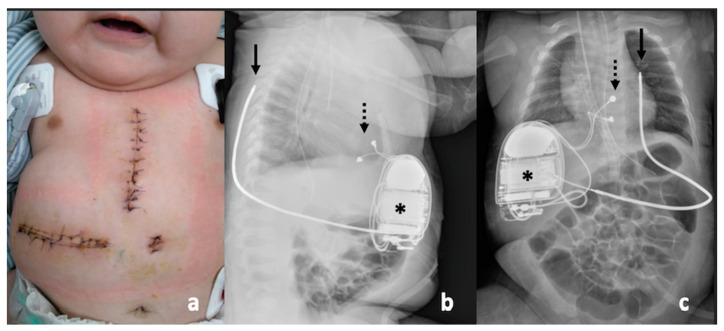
(a) Photo showing the postoperative wound after ICD implantation. (b) Chest X-ray, lateral view. The generator (asterisk) was implanted in a pocket in the right posterior rectus sheath. The subcutaneous defibrillator coil was located in the left subscapular position (arrow). The bipolar epicardial pacing leads (dotted arrow) were placed on the surface of the right ventricle. (c) Chest X-ray, anteroposterior view.
